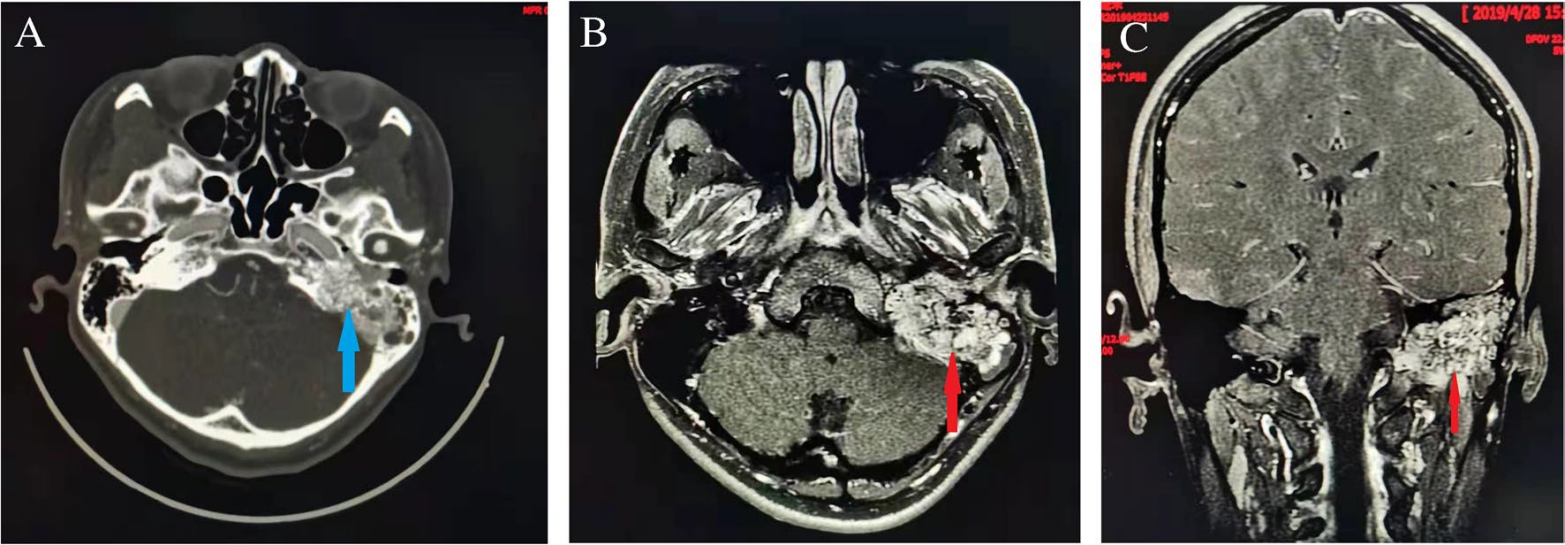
A tympanojugular paraganglioma at the left jugular foramen. A Axial CT scan. B, C Axial (B) and coronal (C) T1-weighted contrast-enhanced MRI. Blue arrow indicates the bony destruction with a moth-eaten appearance. Red arrows indicate the tumor with a salt-and-pepper appearance.
